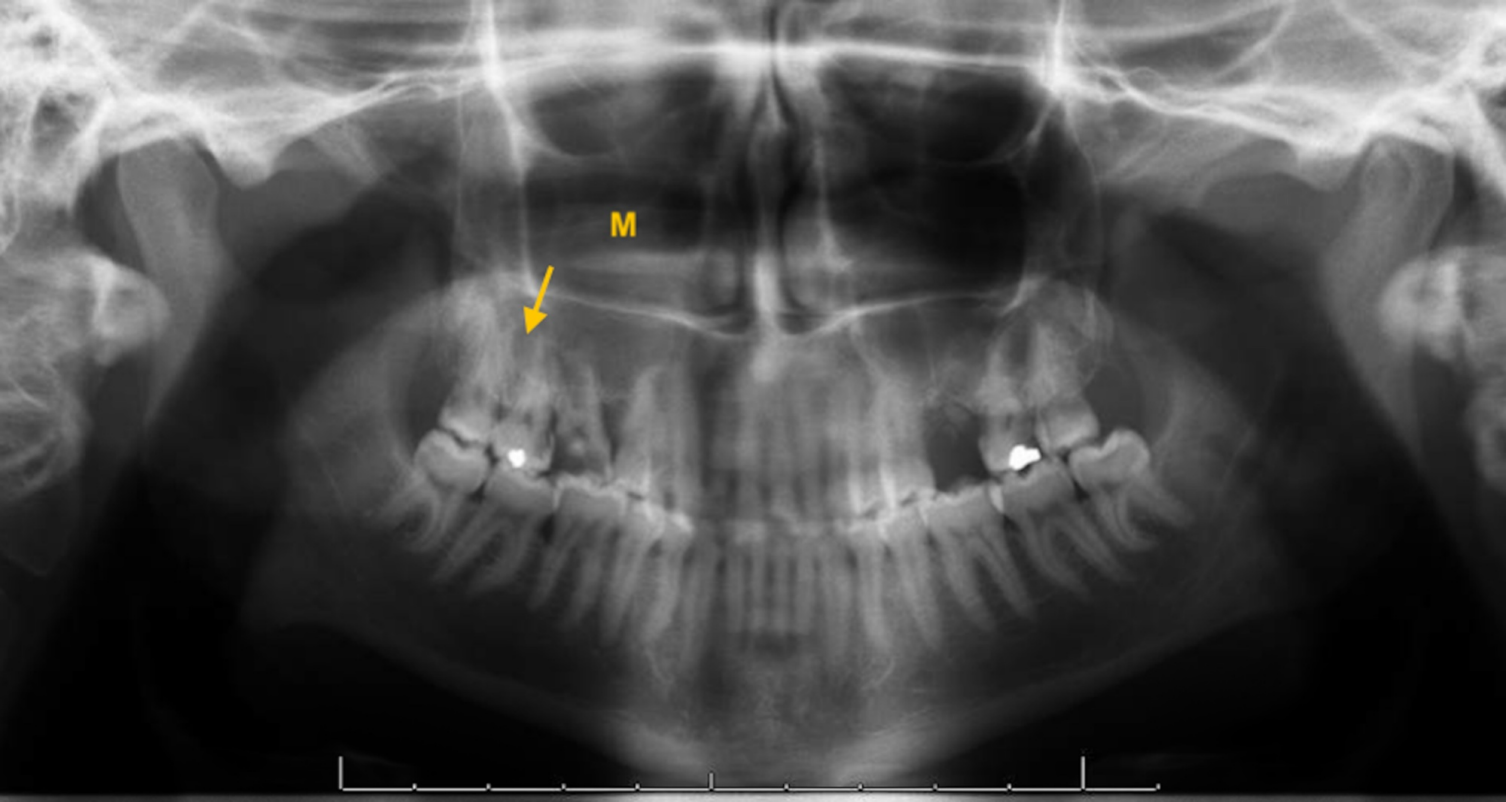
Tooth decay and periapical radiolucency (arrow) associated with maxillary right first molar with opacification of the right maxillary sinus (M) on the panoramic radiograph (orthopantomogram).
